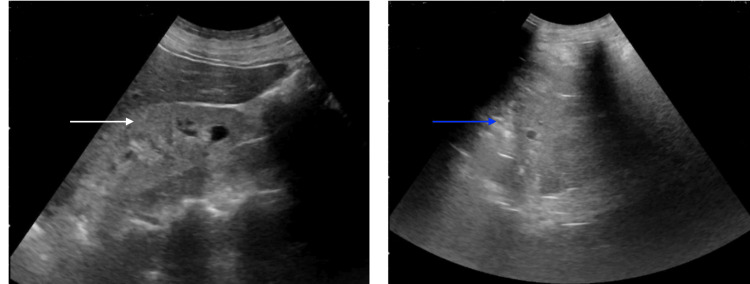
Ultrasound of the bilateral kidneys. White arrow: Right-sided kidney with echogenicities; Blue arrow: Left-sided kidney with echogenicities.
